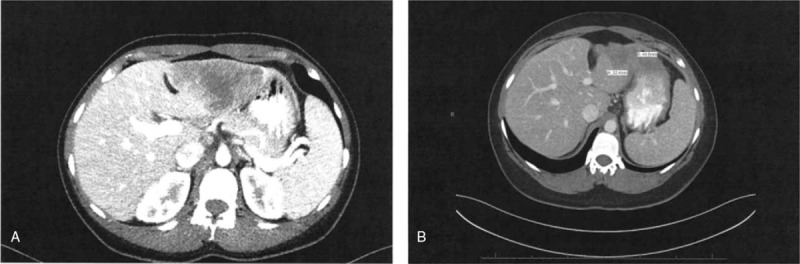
A. Abdominal computed tomography (CT) in July 2015 showed a 9.3 × 4.5 cm liver mass; B. Abdominal CT in April 2016 showed that the liver mass had reduced in size to 3.2 × 4.5 cm.
